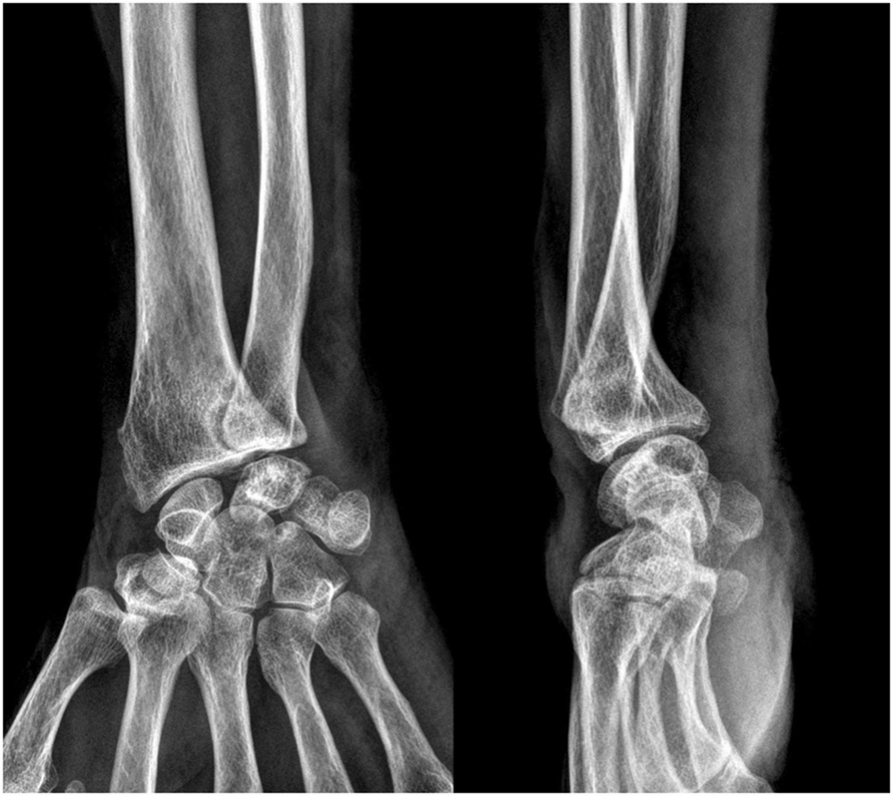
Plain radiographs show osteoarthritic change at the distal radioulnar joint with deepening and widening of the sigmoid notch.
